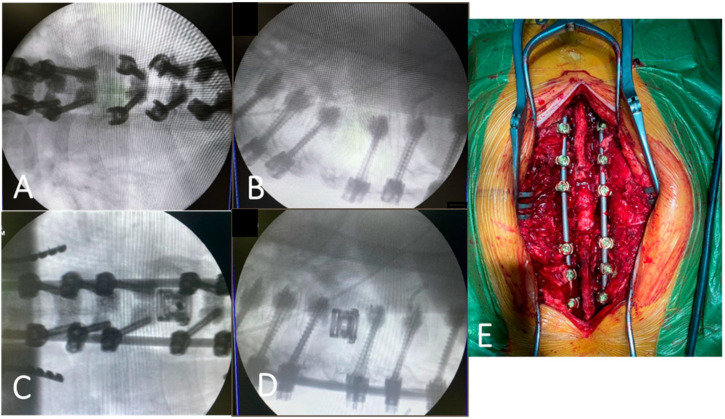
Intraoperative X-rays of implant placement (A–D) and intraoperative imaging (E). (A) Frontal radiograph after transpedicular screw fixation. (B) Sagittal radiograph after transpedicular screw fixation. (C) Frontal radiograph after cage replacement. (D) Sagittal radiograph after cage replacement. (E) Posterior intraoperative view.
